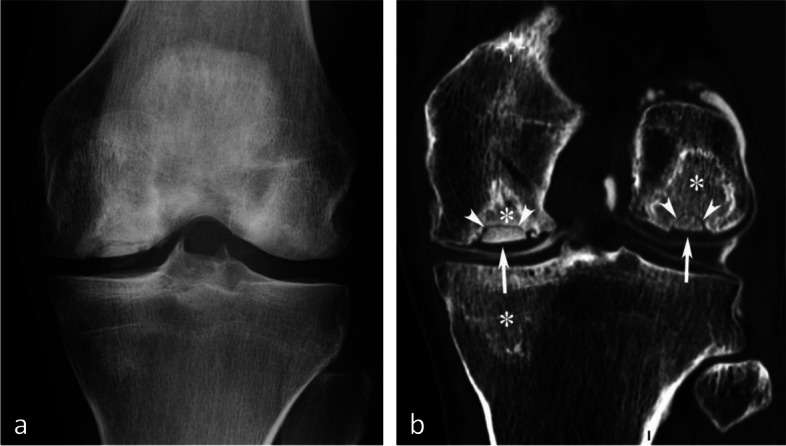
Radiographic of osteonecrosis of systemic origin. a Radiograph shows areas of heterogeneous bone sclerosis, in this case particularly prominent in the condyles. b CT arthrography shows areas of irregular bone densification corresponding to osteonecrotic lesions (asterisks) surrounded by sclerotic rims. Note collapsed segments in the weight-bearing areas of inferior condylar poles (arrows) associated with fractures within necrotic areas (arrowheads)
